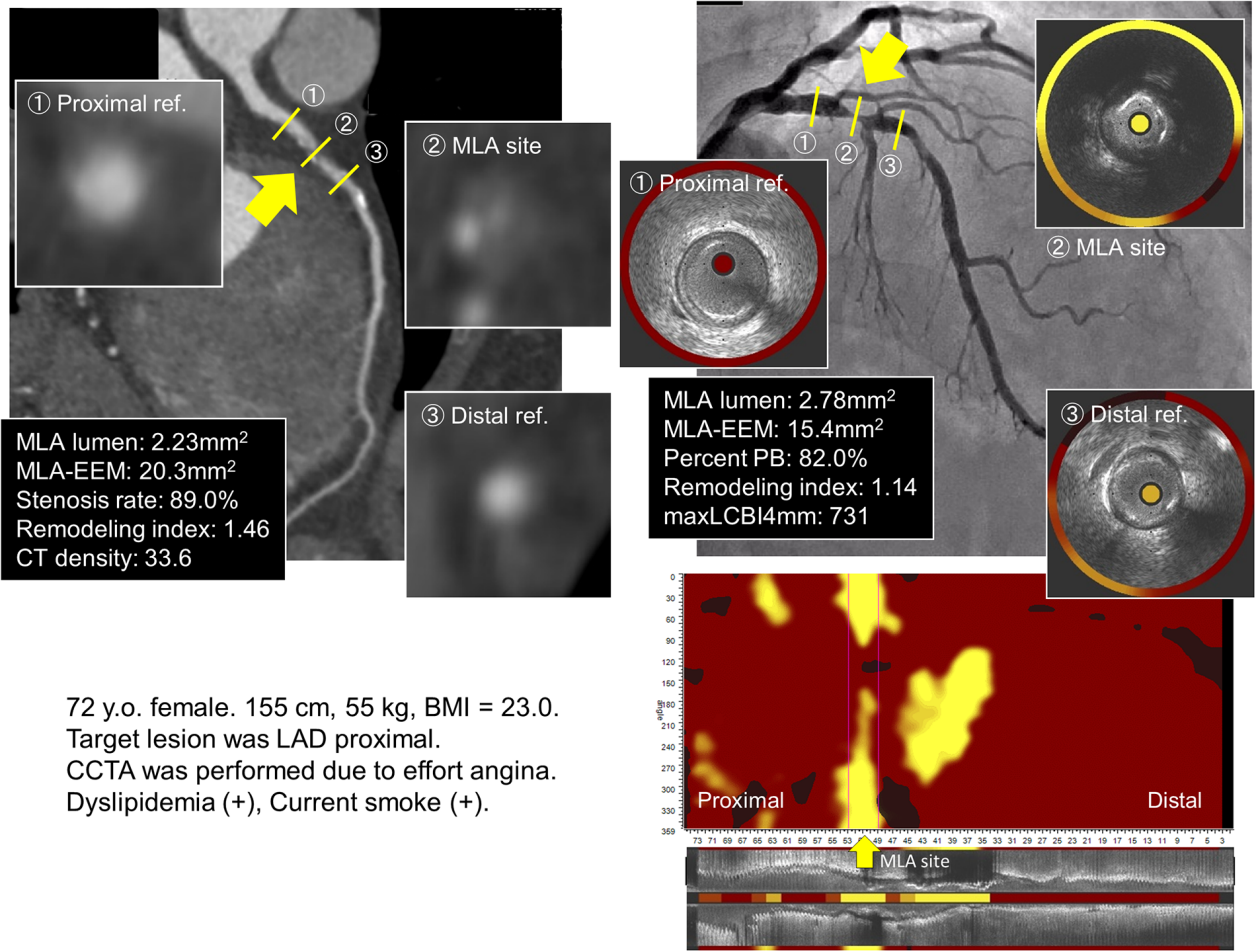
Coronary angiography, CCTA, gray-scale IVUS, and NIRS for the lipid-rich plaque group. The culprit lesion is located at the proximal LAD. CCTA revealed low CT density with positive remodeling; similarly, NIRS–IVUS showed high maxLCBI4mm with positive remodeling in the culprit segment. (The yellow arrow indicates the site of the minimal lumen area.) The cTnT level increased from 0.002 ng/ml to 0.124 ng/ml after PCI. CCTA, coronary computed tomography angiography; IVUS, intravascular ultrasound; NIRS, near-infrared spectroscopy; LAD, left anterior descending; maxLCBI4mm, maximum value of the lipid-core burden index for any 4-mm segment; cTnT, cardiac troponin T; PCI, percutaneous coronary intervention.
